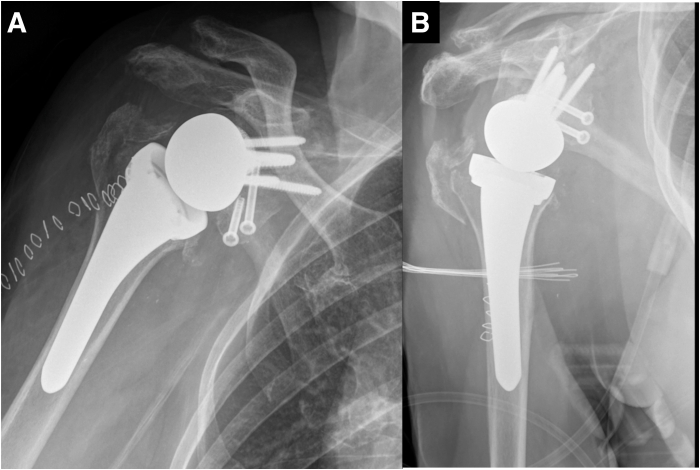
Postoperative anteroposterior (A) and lateral (B) radiographic views of the right shoulder, demonstrating the final position of the baseplate, screws, coracoid graft, and posterior tricortical iliac crest autograft.
